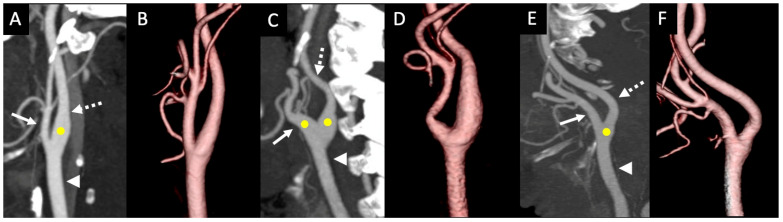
Types of carotid sinus using CT maximum intensity projection images and CT 3D reconstructions: (A,B) type I with dilatation of the CS extending from the distal CCA to the ICA; (C,D) type II with a dilated CCA extending to both the ICA and ECA; (E,F) type III CS with the dilatation in the distal CCA without extension to the ICA or ECA. (Arrowhead = CCA, solid arrow = ECA, dashed arrow = ICA, yellow circle = extension of the CS).
